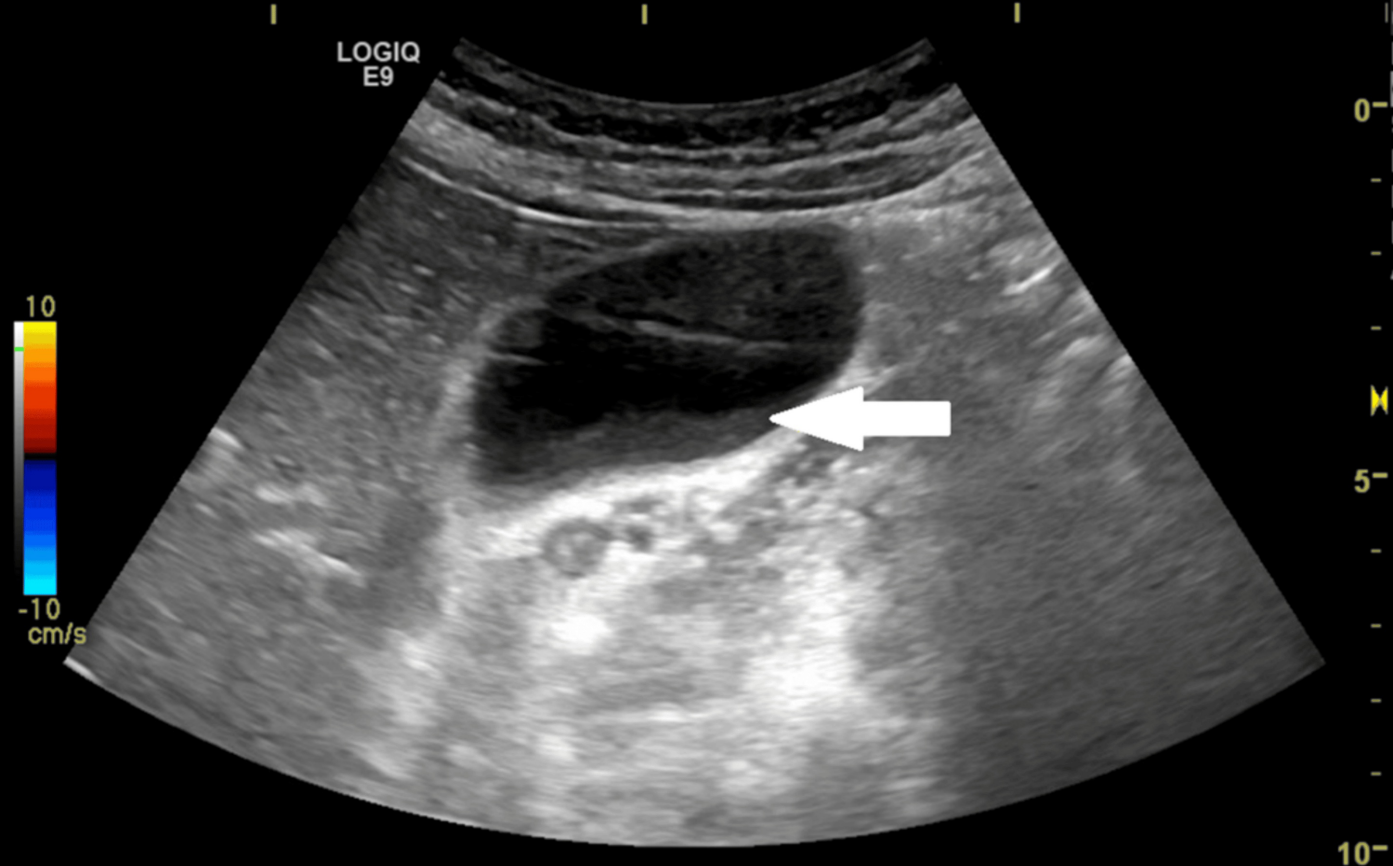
Alternate ultrasound angle confirming the absence of cholelithiasis An additional ultrasound view of the gallbladder (white arrow), further demonstrating the absence of gallstones or biliary tract dilation.
